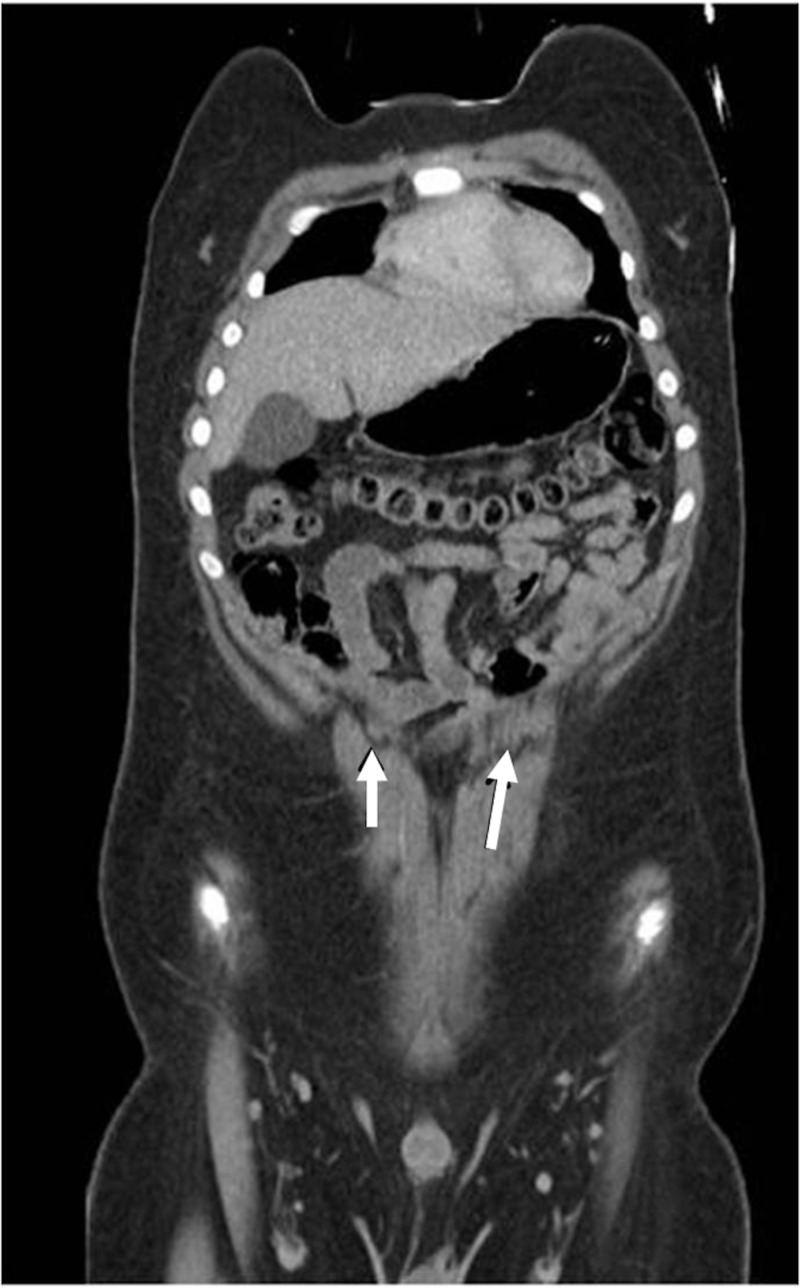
MRI of the anterior abdominal wall. Note the tendinous intersections (arrows). MRI: magnetic resonance imaging
